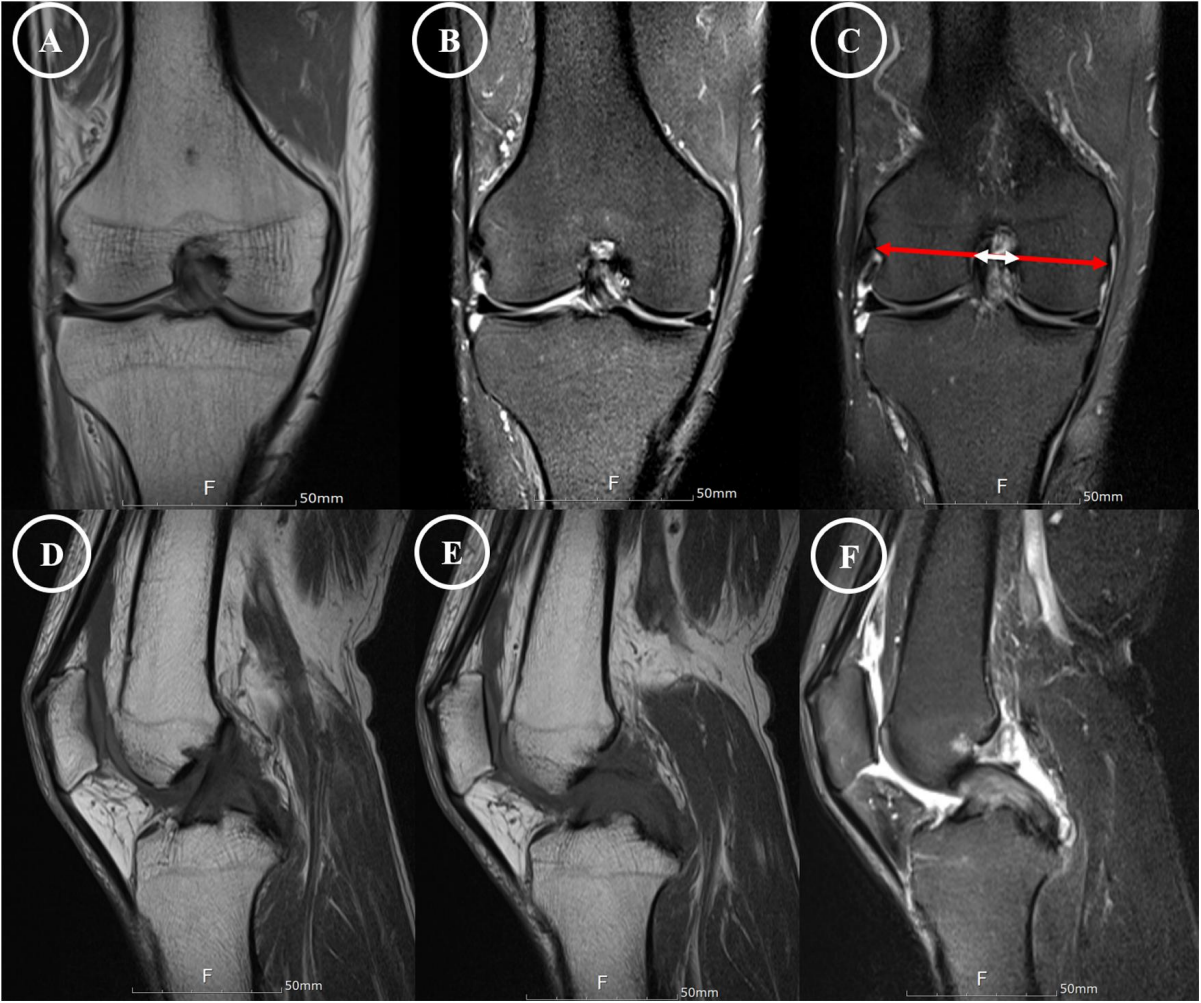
MRI demonstration of mucoid degeneration affecting both cruciate ligaments. (A–C) Coronal proton-density and T2-weighted images showing a markedly narrowed intercondylar notch (notch width ratio of 0.21) with close abutment between the affected ligaments. (D–F) Sagittal views depict the anteroposterior extent of the pathology. In both imaging planes, annotation arrows highlight the characteristic “celery stalk” sign—fusiform hypertrophy and diffuse high signal intensity within both ACL and PCL.
